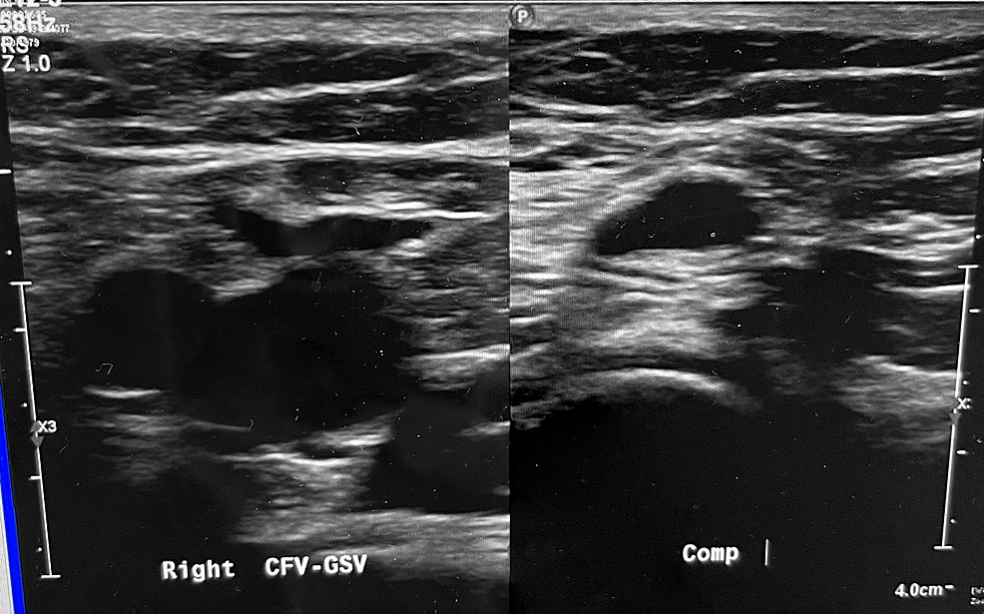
Doppler US Without (left) and With Compression (right) Suggesting Venous Thrombosis
